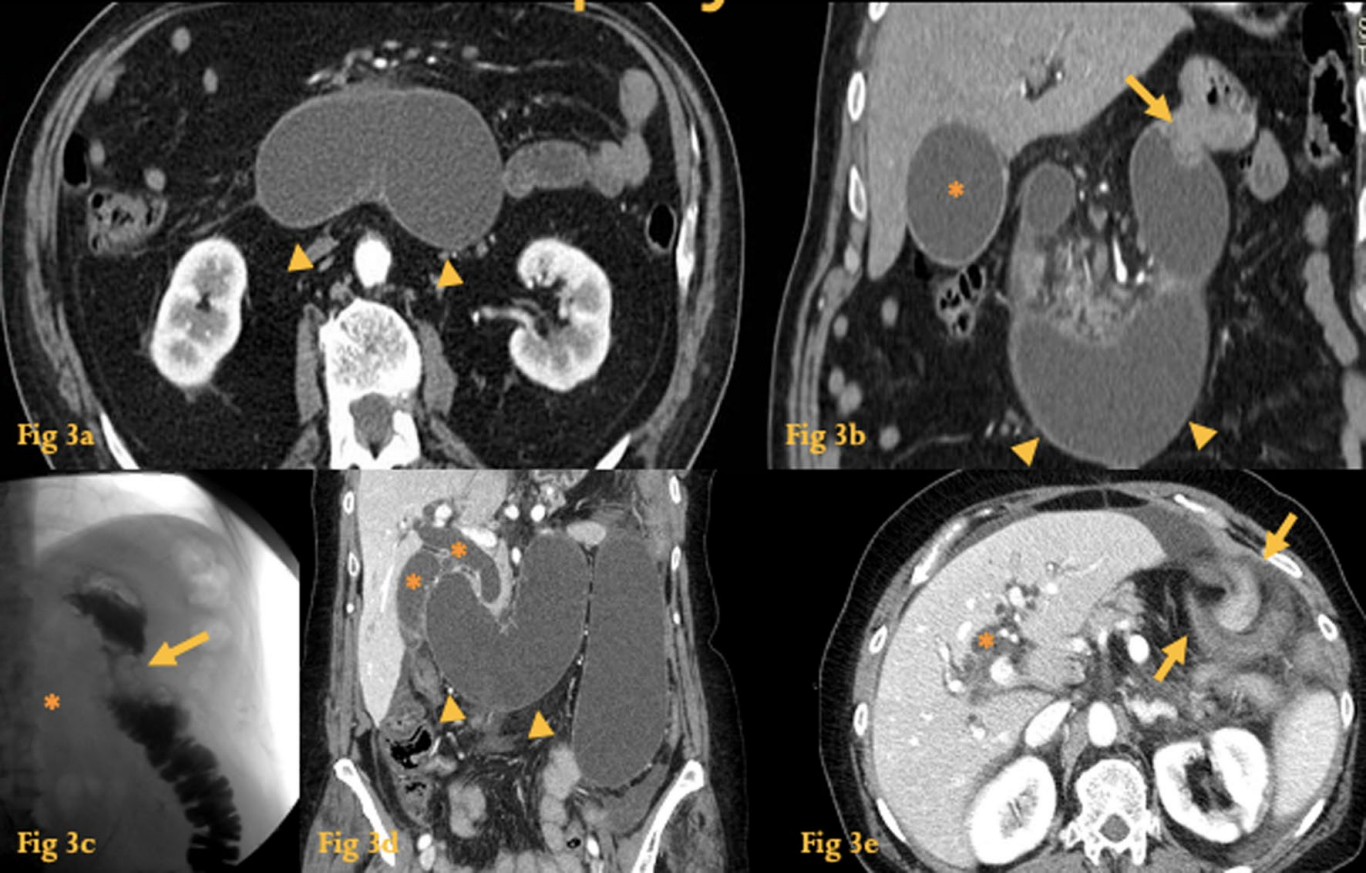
Afferent Loop Syndrome. Abdominal CT (a, b) of a patient who had received partial gastrectomy with Billroth II reconstruction—A fluid-filled tubular structure in the right upper quadrant and crossing the midline is seen, with associated gallbladder distension (asterisk). There is an enhancing lesion at the anastomosis. This was an afferent loop syndrome caused by gastric tumor recurrence. UGI series of the same patient (c). There is non-filling of the afferent loop (asterisk) and a filling defect at the location of the anastomosis (arrow). Abdominal CT (d, e) of another patient with afferent loop syndrome, because of an internal hernia with volvulus (note the twisted configuration of the bowel loops at the left hypochondrium (arrows)). There is fluid-filled dilatation of the afferent loop associated with gallbladder distension and intrahepatic and extrahepatic duct dilatation (asterisks)
